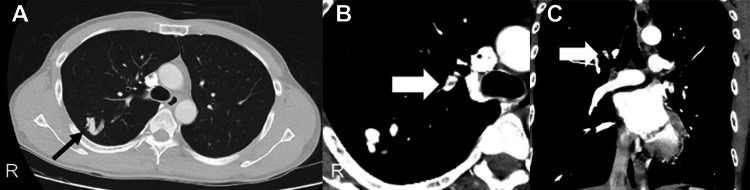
Computed tomography scan of the chest. (A) A pulmonary arteriovenous fistula with a dilated draining vein is observed on the right S2 (black arrow). The contrast-enhanced computed tomography scan shows the thrombus (white arrow) in the feeding artery. (B) Axial view. (C) Coronal view.
